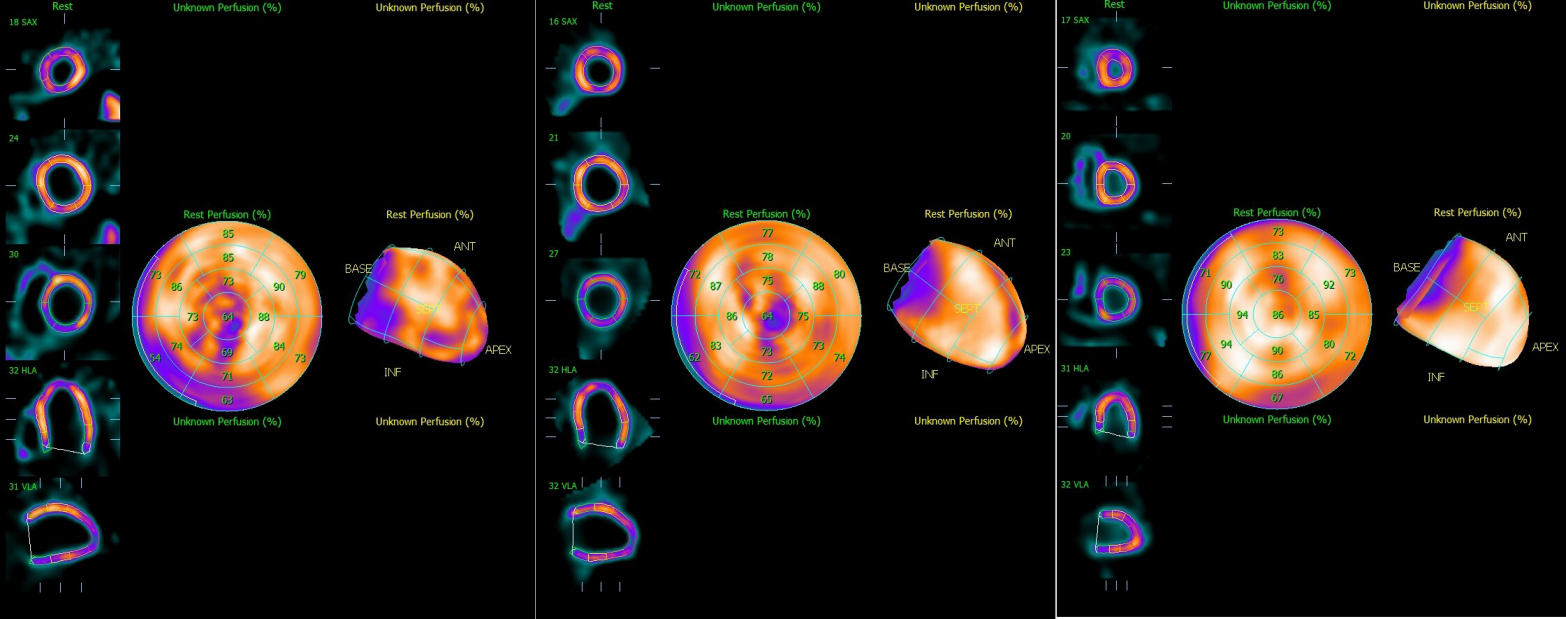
Representative Single-Photon Emission computed tomography images of (A) Total ischemia, (B) Part ischemia, and (C) Normal.
